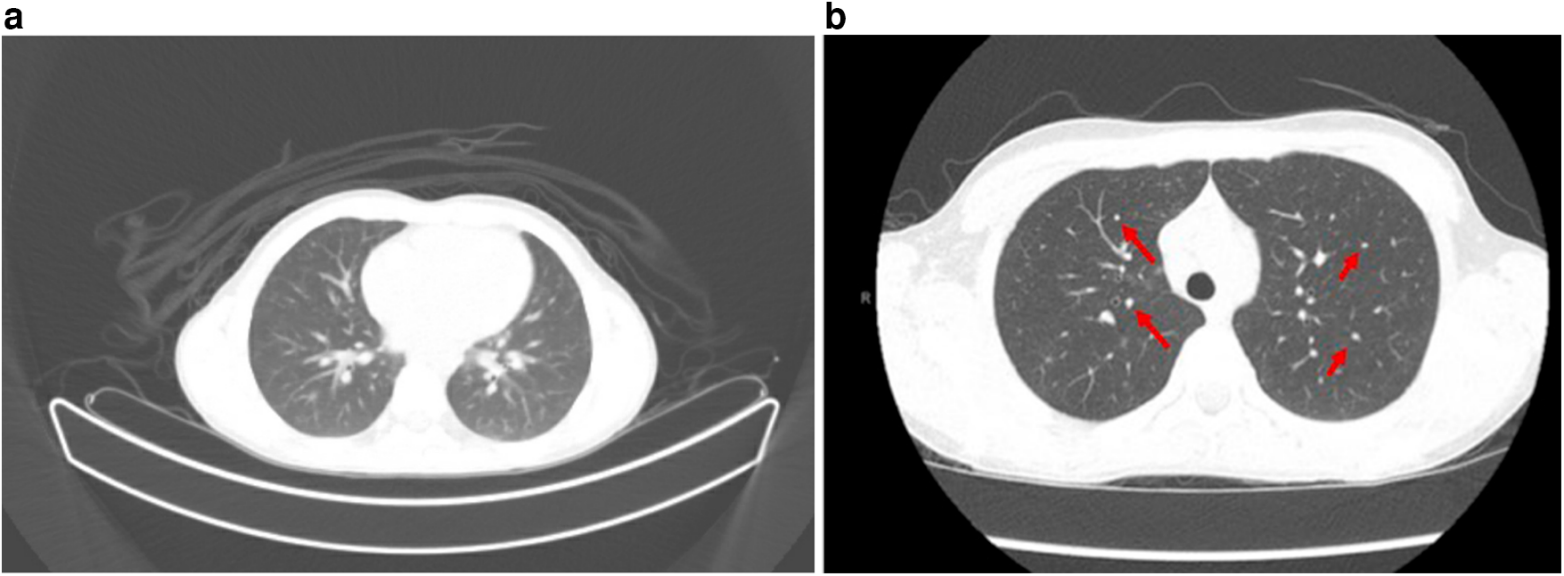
(a) PET scan with multiple mediastinal, axillary, and common hilar lymph nodes are appreciated, with representative lymph nodes. Multiple ground glass nodules within the bilateral lungs predominantly in a centrilobular distribution. Additional indistinct ground glass opacities along the posterior aspect of the bilateral lung. (b) CT scan with enlarged mediastinal lymph nodes demonstrated, correlating with hypermetabolic nodes from PET above. Multiple non-specific subcentimeter ground glass nodules (red arrows) throughout both lungs.
